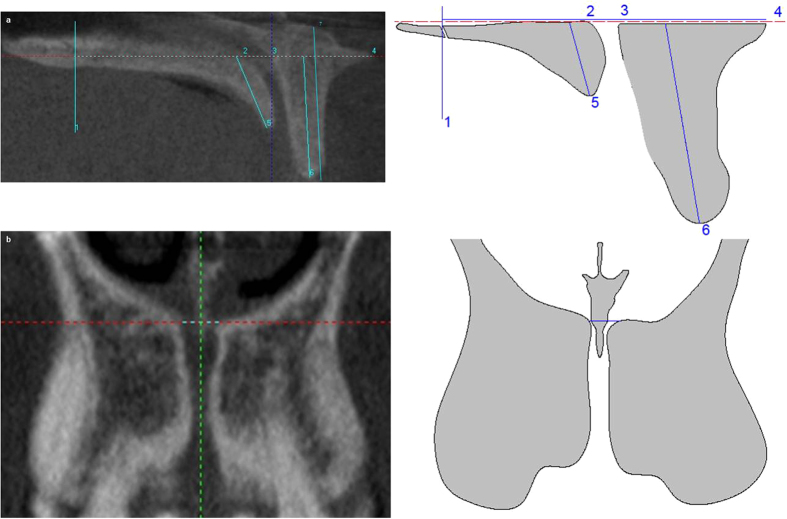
Radiographies and draw- scheme of level 3 show the follows: (a) Sagittal slice: (1) the most posterior point of the transverse palatine suture, (2) PW3, (3) SD3, (4) BW3, (5) PL3, (6) BL3 and (7) tBL. (b) Coronal slice: CD3.
